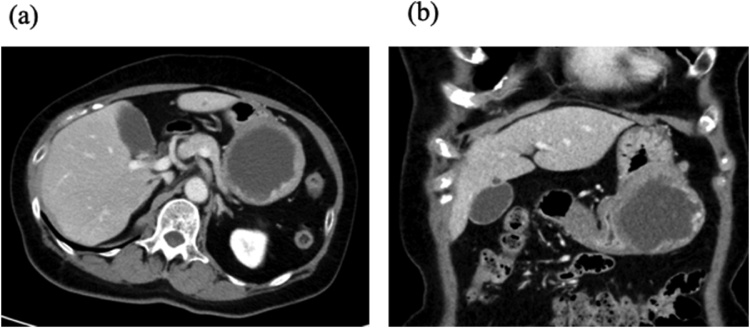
Enhanced abdominal computed tomography at the onset of abdominal pain. a/b. The gastric tumor had moved to the side of the greater curvature and the stomach was twisted. There is no evident contrast failure area of the stomach.
